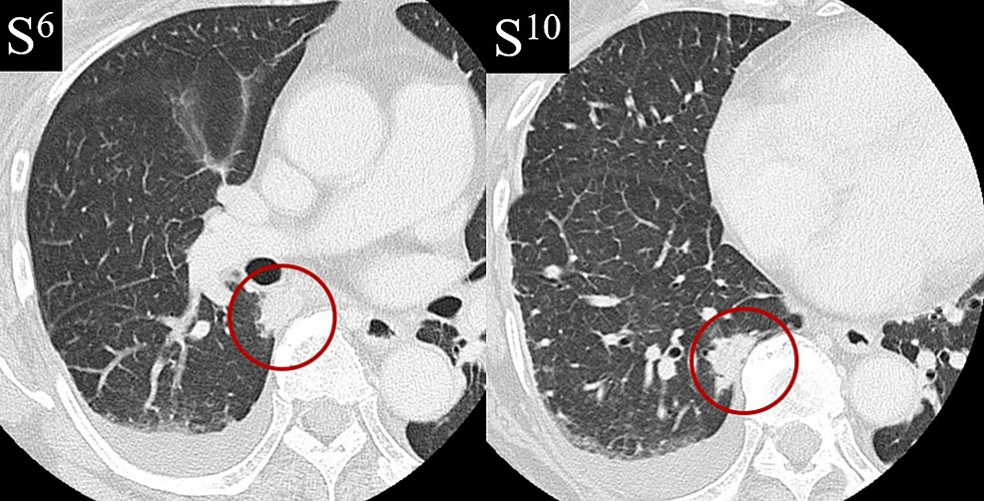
Contrast-enhanced CT revealed no source of fever other than two mass lesions (18×11 mm and 26×10 mm) in S6 and S10 in the right lower lobe. Pleural effusion, which may be influenced by inflammation, was also observed in the right lung.
